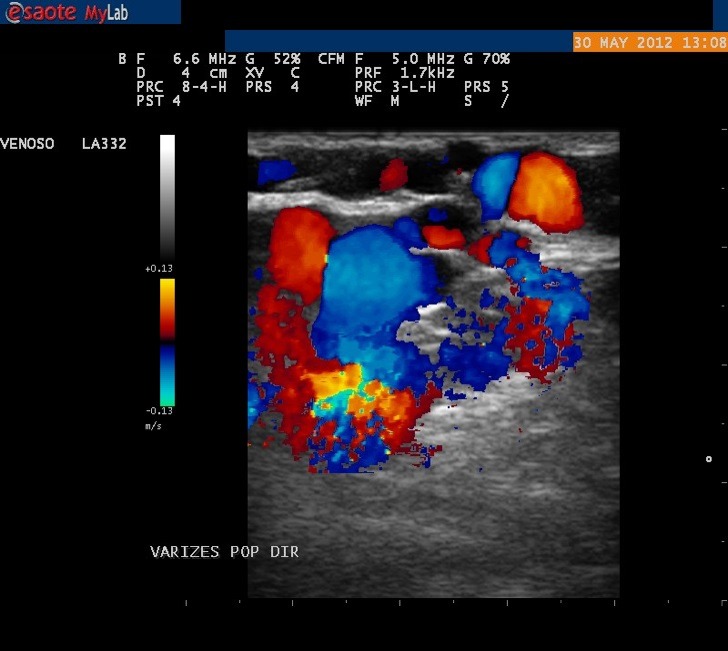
Ultrassom Doppler evidenciando varizes com distribuição atípica em membro inferior direito.
